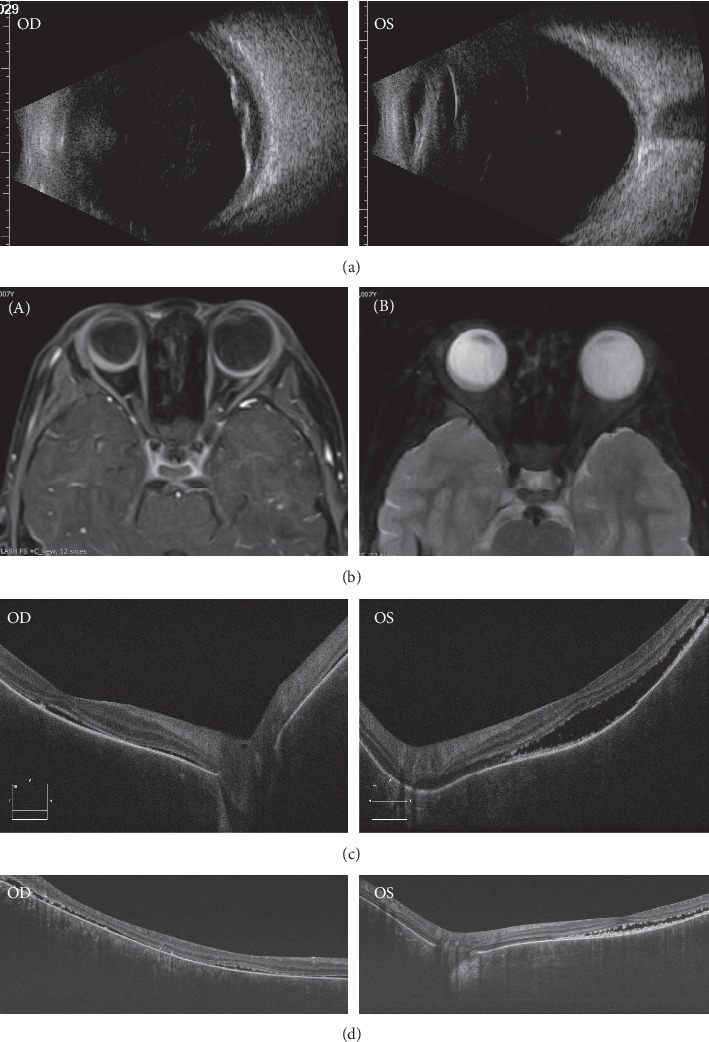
Noninvasive cross section images of the fundus. (a) Ultrasound B scan showed diffuse thickening with the middle strength signal in choroidal layer bilaterally. Secondary retinal detachment was observed in the right eye. (b) MRI showed smooth, diffuse thickening of the eyewall bilaterally: (A) axial, postcontrast fat saturated T1-weighted image; (B) axial, fat saturated T2-weighted image. (c) SD-OCT showed extensive retinal detachment bilaterally. (d) SS-OCT showed low reflections in choroid, revealing the absence of choroidal the vasculature.
